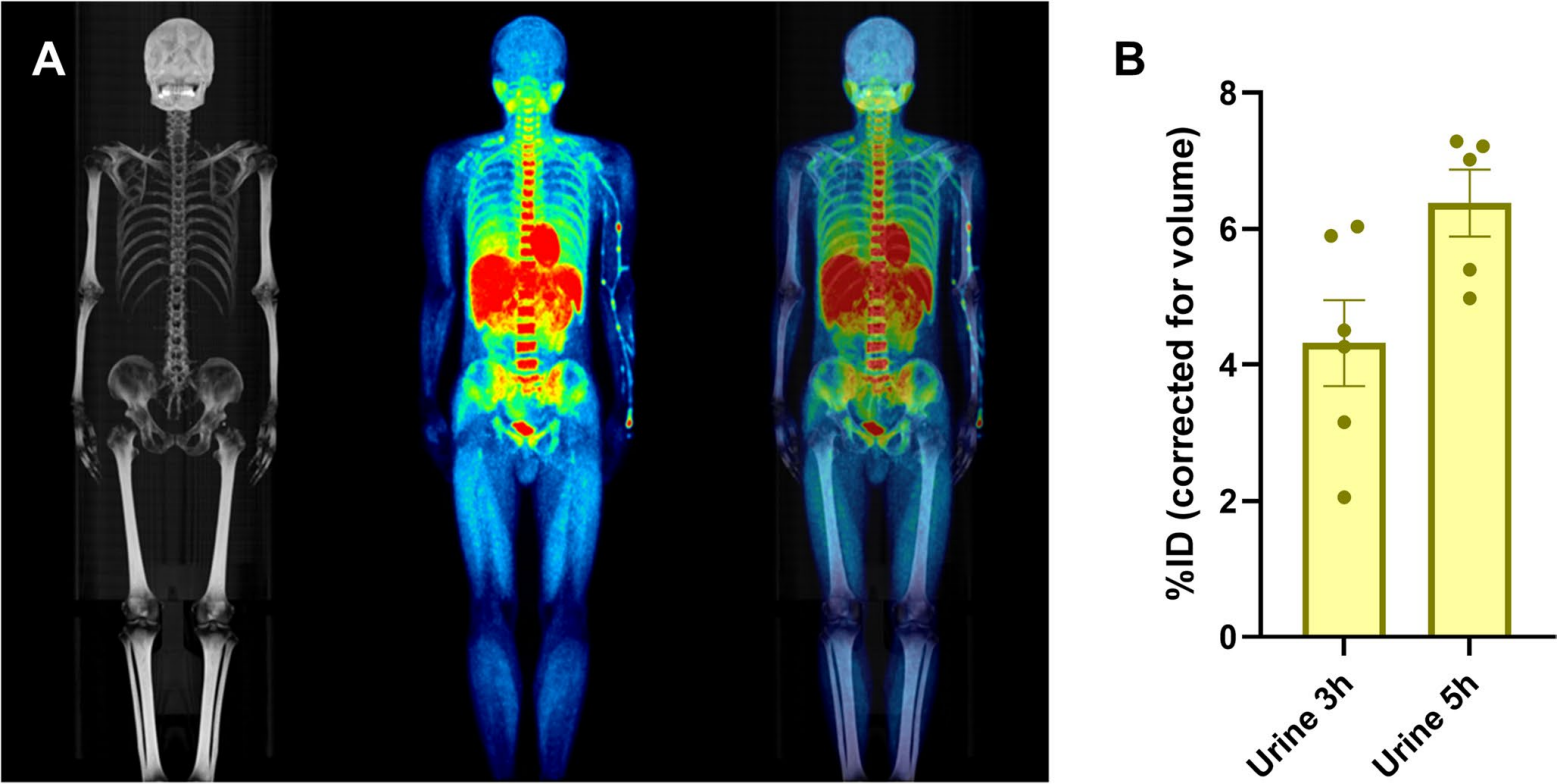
[^18^F]LW223 in vivo distribution and urinary excretion. (A) Maximum intensity projection (MIP) CT image, decay-corrected whole-body PET maximum intensity projection SUV average image (0–60 min) of [^18^F]LW223 distribution in an adult human male and overlay of CT and PET MIPs. (B) Percentage injected dose (%ID) corrected for total urine volume void up to 5 h post-radiotracer injection. Data presented as mean ± SEM, *n* = 5–6
